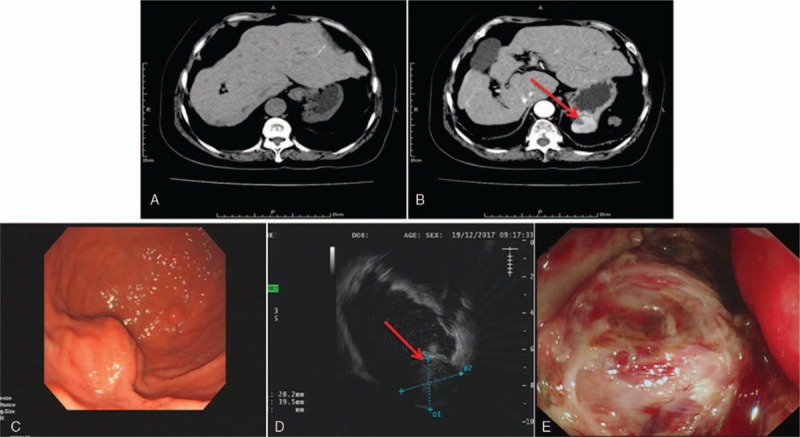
(A) CT scan shows pipe stem cirrhosis. (B) CT scan reveals an approximately 4-cm mass in the gastric fundus wall with a homogeneous contrast enhancement, which is typical in GISTs, and a 1-cm low-density solid lesion with a clear outline within the mass (red arrow). (C) Gastroscopy shows a mass in the gastric fundus. (D) Endoscopic ultrasonography reveals a 3.95 × 2.82-cm slightly low echoic mass with a homogeneous appearance in the muscularis propria of the stomach fundus wall and a low echoic area with a clear boundary in the mass (red arrow). (E) The mucosa and submucosa were cut open, and the mass was exposed in the muscularis propria of the stomach fundus wall. The surface of the mass was covered by larger blood vessels. CT = computed tomography, GIST = gastrointestinal stromal tumor.
